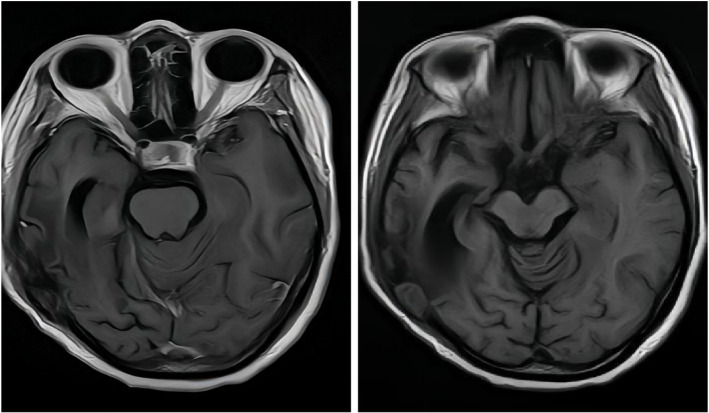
After Six cycles of chemotherapy and radiotherapy, completion of treatment confirmed complete disease remission through T1-weighted sagittal MRI.
